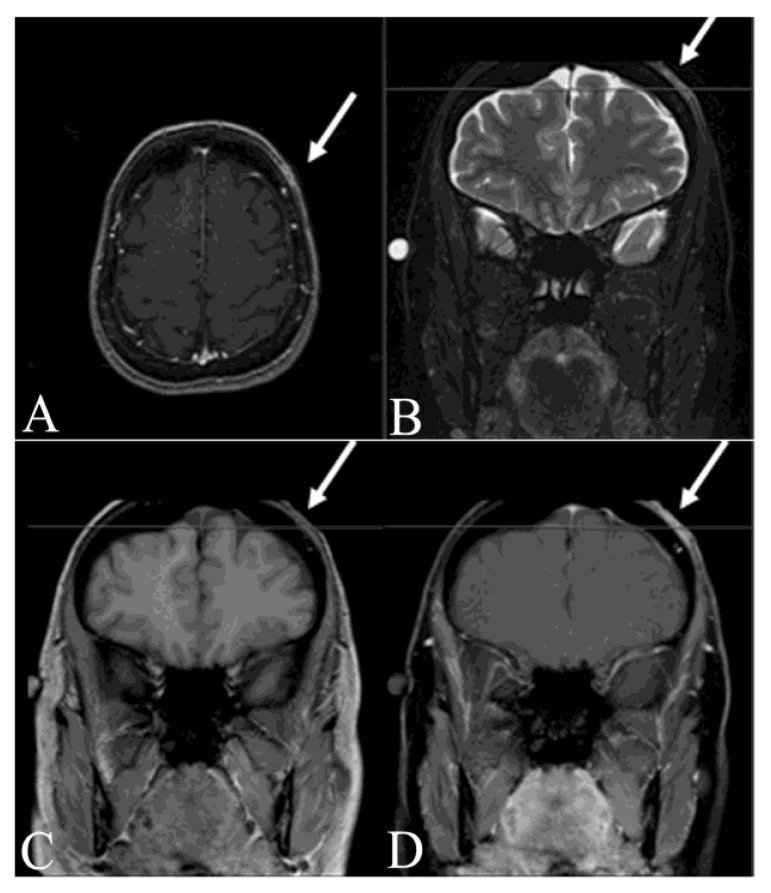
Magnetic resonance imaging of fibrosarcomatous-dermatofibrosarcoma protuberans on the left forehead from patient in Figure 1. (A) Left scalp enhancement (arrow) in axial post-gadolinium thin slice image; (B) left scalp hyperintensive lesion without bony invasion (arrow) on coronal fat saturated T2-weighted image; (C) left scalp isointense lesion (arrow) before gadolinium on T1-weighted image; (D) left scalp hyperintense lesion (arrow) after gadolinium on T1-weighted image.
